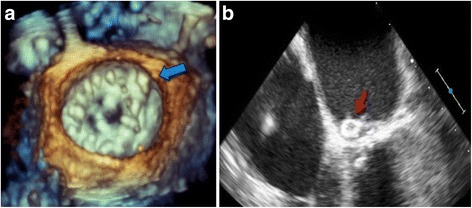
Intra-procedural Trans-esophageal echocardiography. a 3-D Zoom view on 3-D TEE post-deployment showing final position of Amplatzer vascular plug II (*blue arrow*). b 4-Chamber view on 2-D TEE showing Amplatzer vascular plug in situ (*red arrow*)
